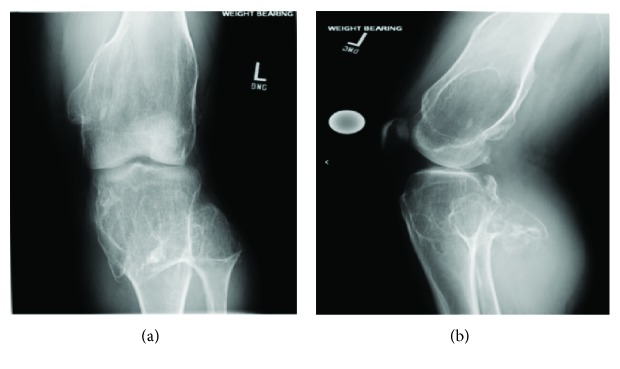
A preoperative anterior-posterior (AP) and lateral X-ray view of the patient's left knee showing significant osteochondroma formation both superior and inferior to the knee joint. Subchondral sclerosis as well as medial and lateral loss of joint space is appreciable.
